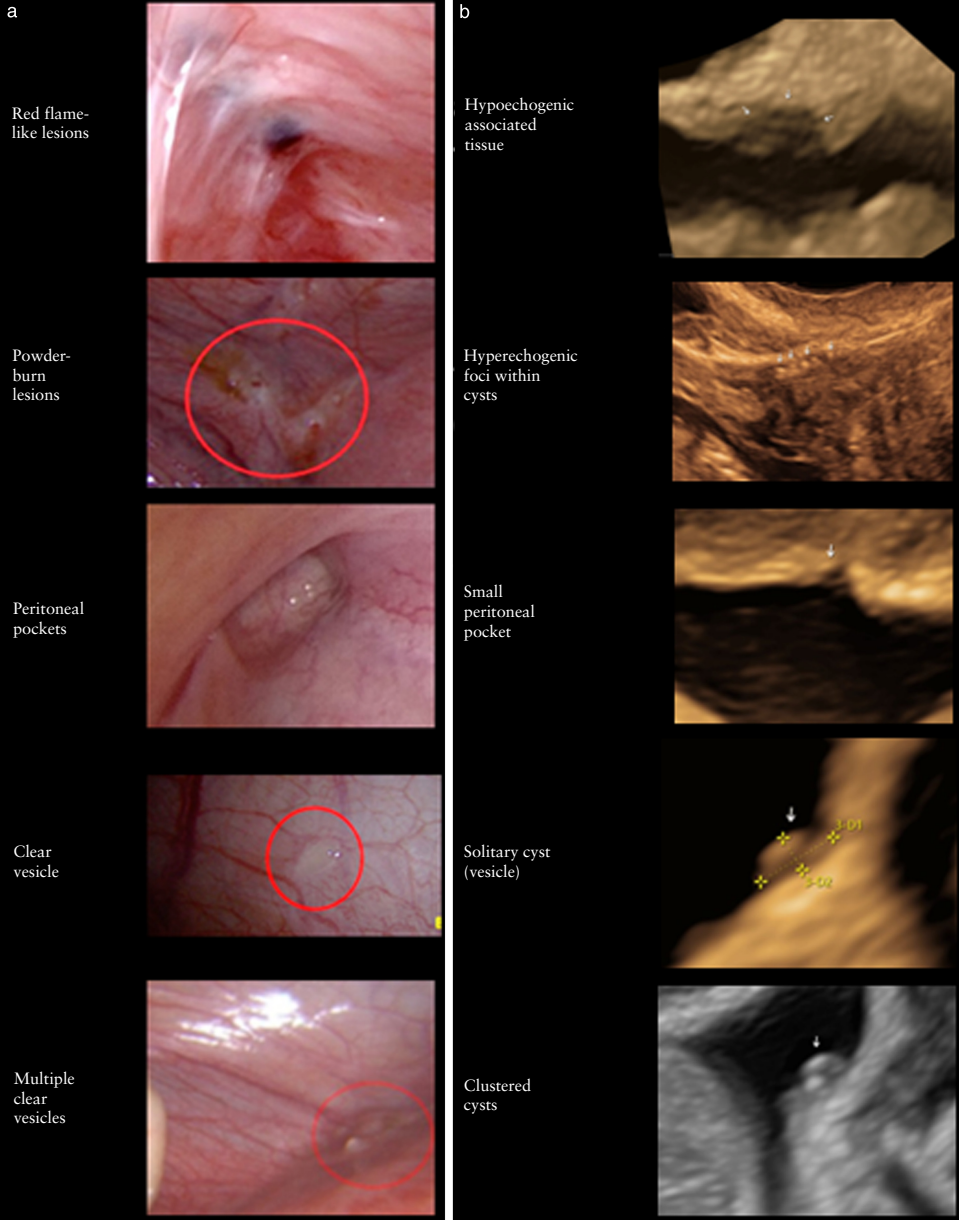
(a) Appearance of superficial endometriosis at laparoscopy (based on Van Langendonckt *et al*.^15^) and (b) corresponding ultrasonographic findings (based on Pedrassani *et al*.^7^, Leonardi *et al*.^16^ and Bailey *et al*.^25^).
